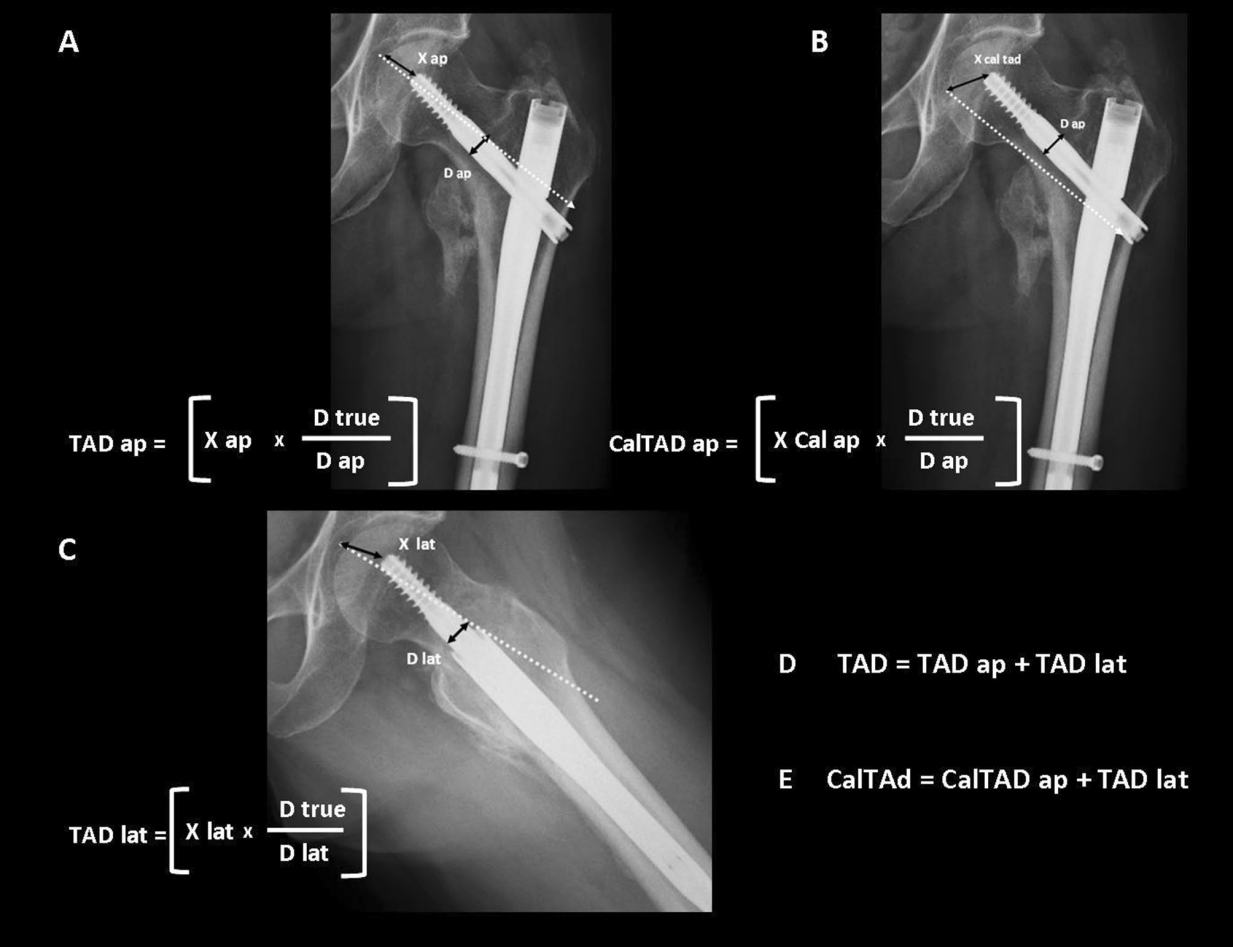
(A) Tip-apex distance calculated on anteroposterior radiograph (TAD ap); (B) Tip-apex distance as referenced to the calcar calculated on the anteroposterior radiograph (CalTAD ap); (C) Tip-apex distance calculated on the lateral radiograph (TAD lat); (D) Tip-apex distance (TAD); (E) Calcar-referenced tipapex distance (CalTAD). D true is the known diameter of the lag-screw (10.5 mm for Gamma3 nail, 15.5 mm for Trigen Intertan nail). D ap is the calculated diameter of the lag-screw on the anteroposterior radiograph. D lat is the calculated diameter of the lag-screw on the lateral radiograph.
